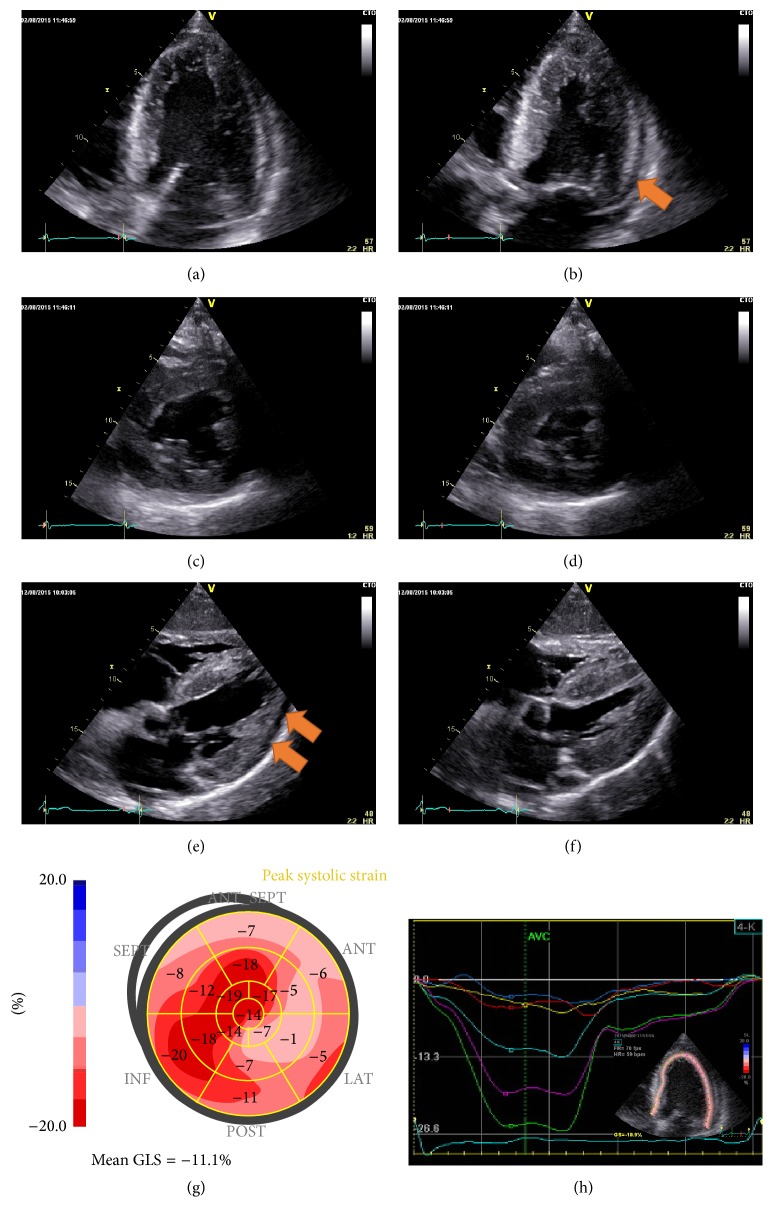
Echocardiographic images revealing severe left ventricular hypertrophy ((a)–(f)). Reduced longitudinal function was noticed in the presence of preserved radial deformation and ejection fraction ((g)-(h)). Minimal pericardial effusion was noticed (orange arrows in (b) and (e)).
